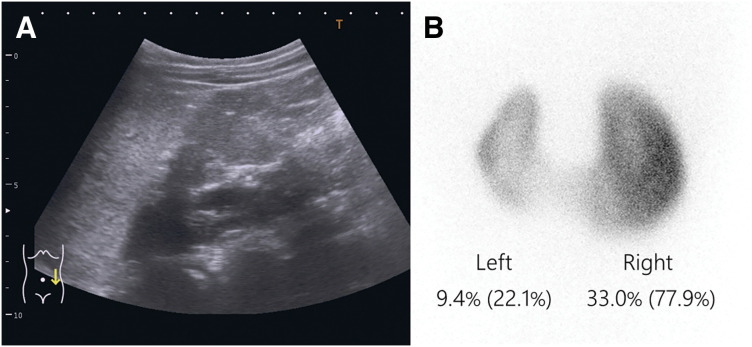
Postoperative examination images. (A) Ultrasonography showed mild dilation of the left renal pelvis (SFU classification grade 1). (B) Renal scintigraphy revealed that the DRF in the left kidney was 22.1%. DRF, differential renal function; SFU, Society for Fetal Urology
